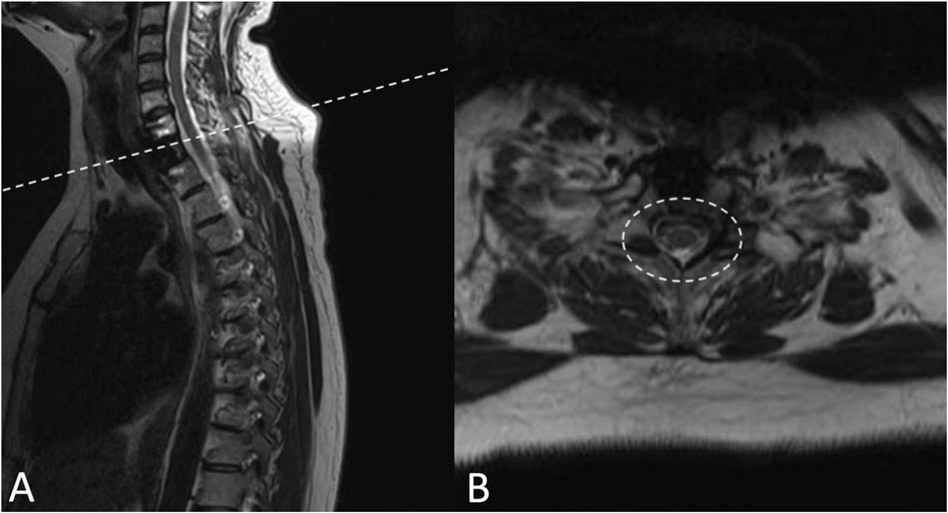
A 15-months postoperative follow up MRI Scan in May 2021. (A) Sagittal view of VBR cage indicating tumour free T2 body. (B) Transverse view of spinal compression reduction and resolved ESCC score.
